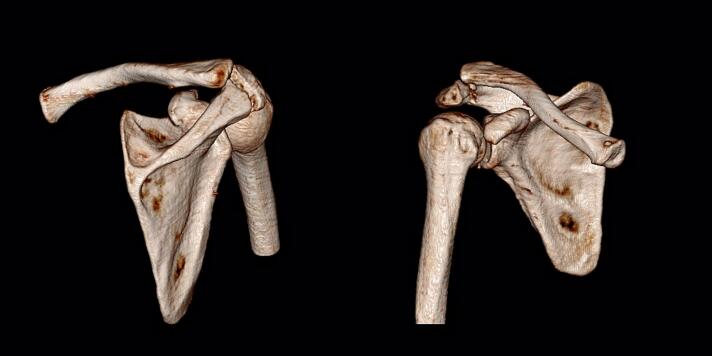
Preoperative CT scan indicating acromion fracture and AC dislocation.
